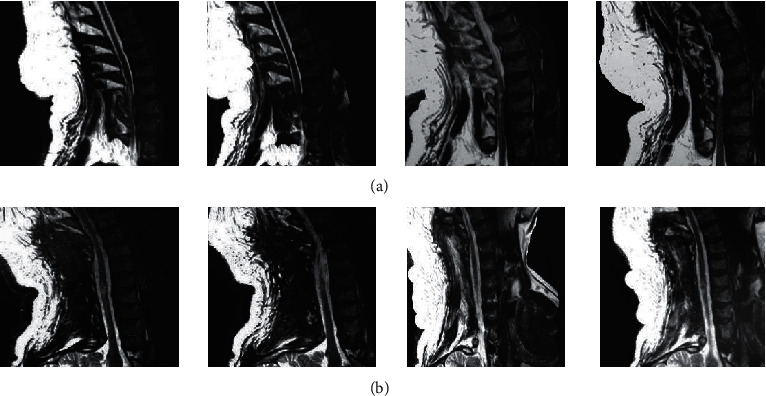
MRI images of a male patient before and after the intervention. The patient was 52 years old, with lumbar pain, numbness, and weakness of both upper limbs due to contusions and was admitted to the hospital for aggravation of walking weakness for 3 months. (a) Before the intervention. (b) After intervention.
